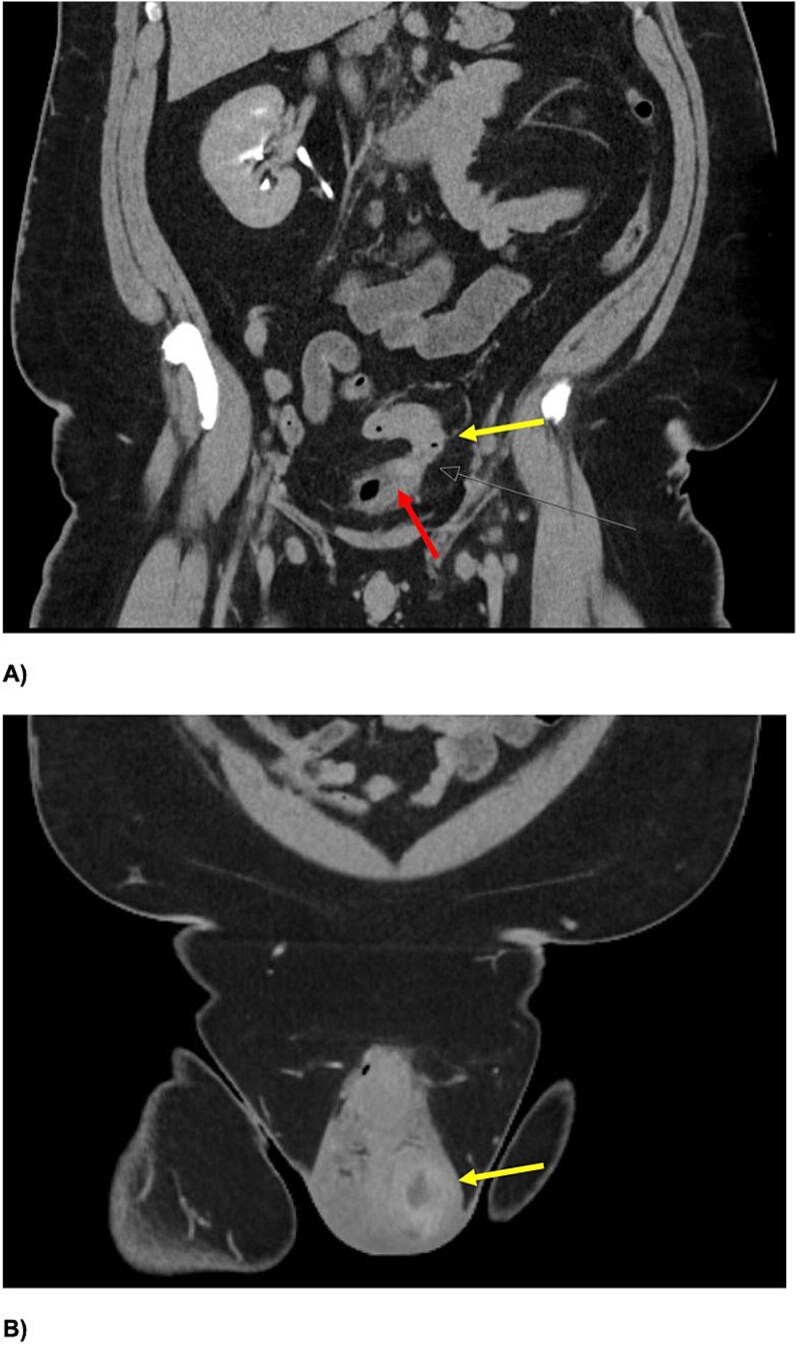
Coronal slices of CT IVP (A) demonstrating a colovesical fistula between the inferior wall of the proximal sigmoid colon (yellow arrow (A)) and superior aspect of the urinary bladder (red arrow). There is intravesical gas with eccentric irregular anterior bladder wall thickening, and mild perivesical fat stranding. An enlarged left testis, with central hypodensity, and left testicular-scrotal abscess is also demonstrated (B).
